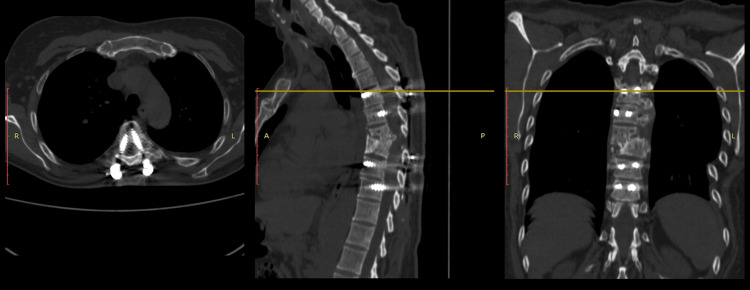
Case 1 CT scan post-surgical images of the T4-T5-T8-T9 transpedicular fixation in the axial, coronal, and sagittal planes.
